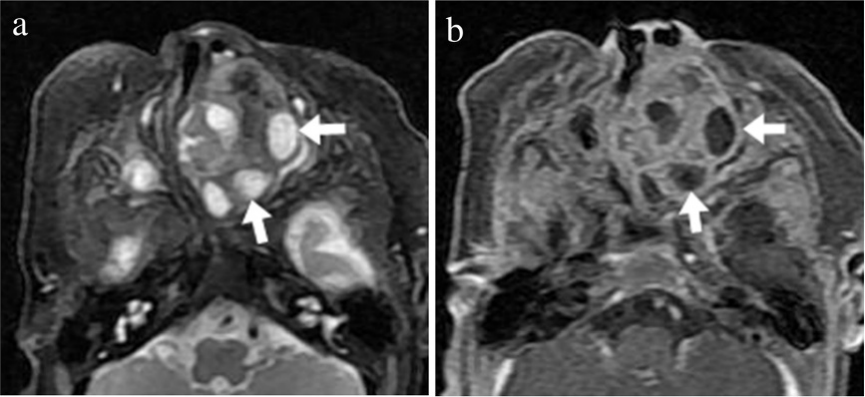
MR Imaging of sinonasal cavity. (a) An axial T2-weighted MRI showed a well-defined, heterogeneous hyperintense mass containing multiple small round areas of higher signal intensity (arrow). (b) Axial contrast-enhanced fat-suppressed T1-weighted image demonstrated striking heterogeneous enhancement of the mass with multiple non-enhanced cystic components within the lesion (arrow).
